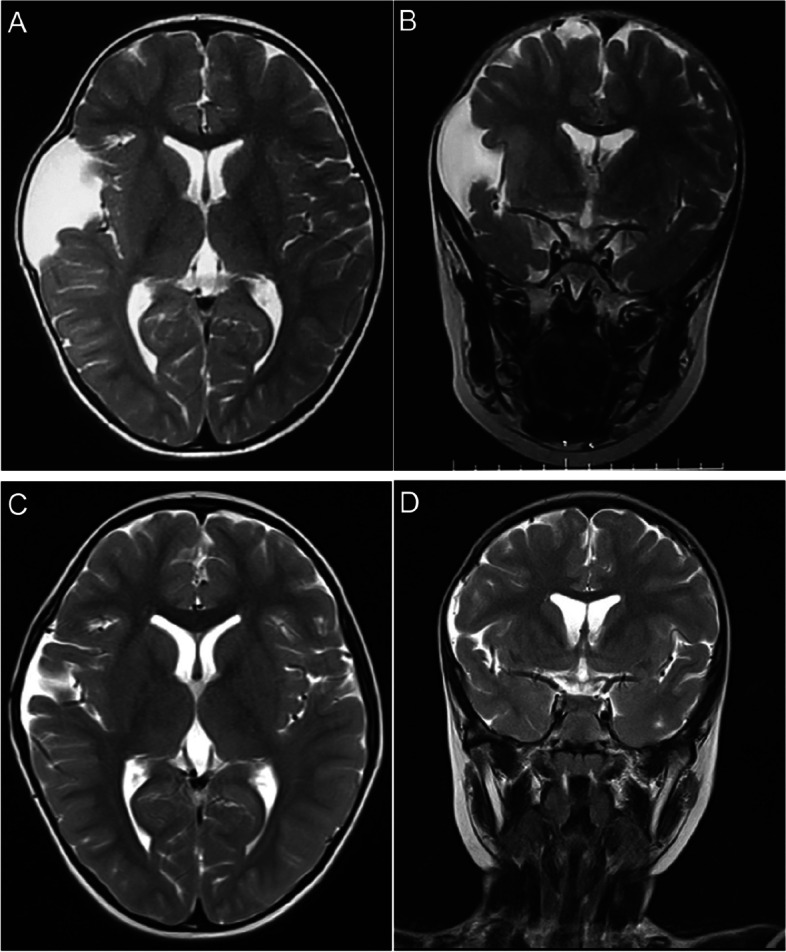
A 2-year-old boy presented with headache and skull deformity. A, B Preoperative axial and coronal T2-weighted magnetic resonance image of the head showing Sylvian AC and bone bulging on the right. C, D Axial and coronal T2-weighted MR image showing total disappearance of the cyst on 24 months follow-up MR imaging
